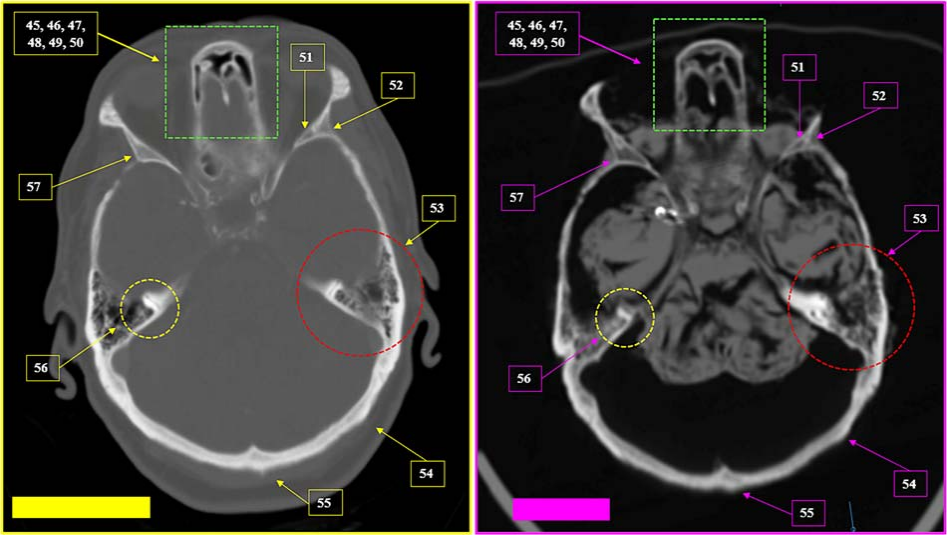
Matching morphology of the frontal sinus ethmoid recess (superior square); multiple coinciding points in the internal table of the skull in the antemortem (left) and postmortem (right) CT scans in axial sections.
